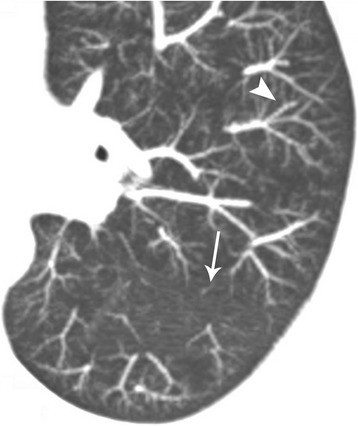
Images from 16-slice spiral computed tomography (CT). The standard algorithm generated transverse images: width of the lung window, 1000 Hounsfield units (HU); window level, − 700 HU. The 12-mm-thick axial maximum intensity projection-rendered image of the upper segment of the *left* major fissure shows decreased density of multiple *shadow lines* and the surrounding structures overlapping with multiple *shadow lines*, with most of the shadow lines being no longer visible (*white arrow*). Segmented small blood vessels are visible (*white arrowhead*)
